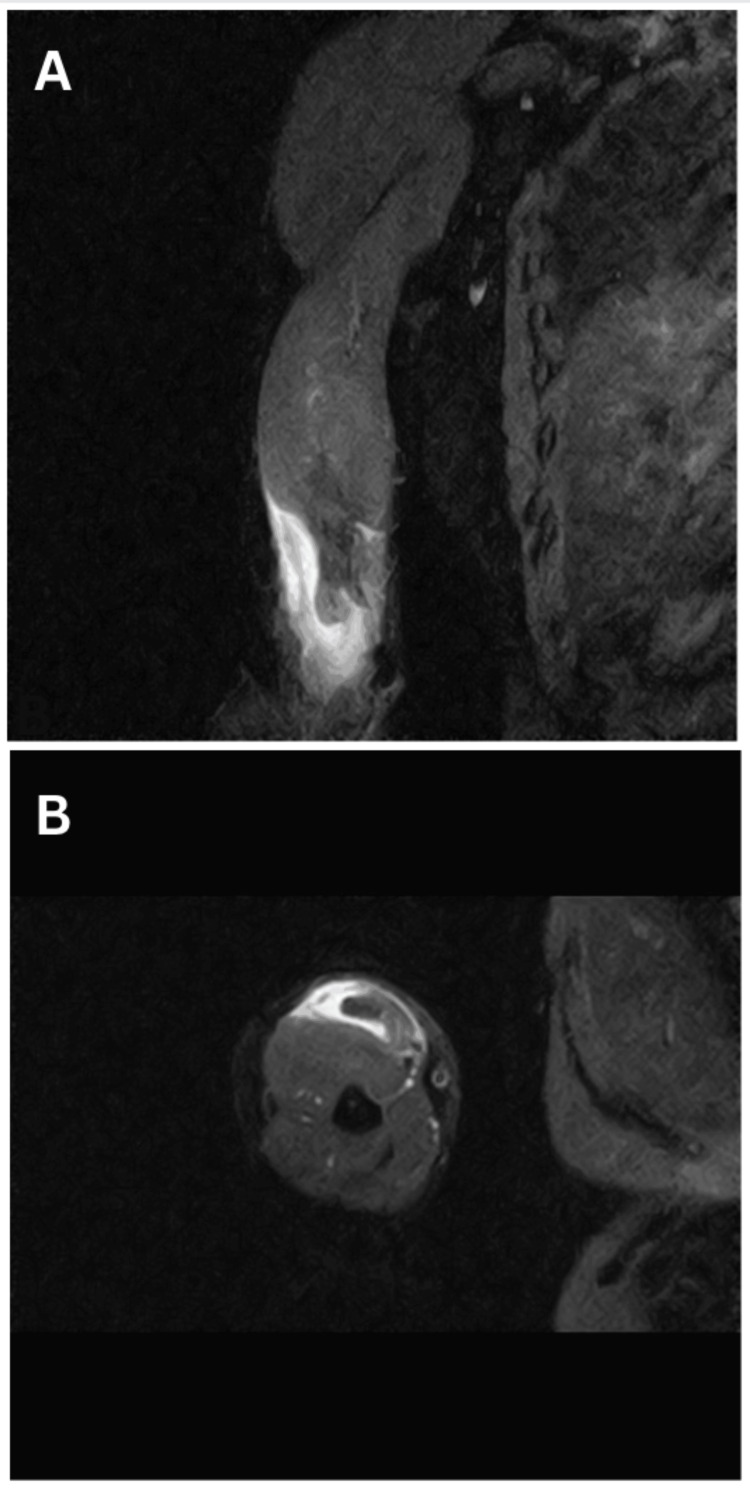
(A) Sagittal MRI image showing distal biceps tendon rupture. (B) Axial MRI image showing distal biceps tendon rupture
